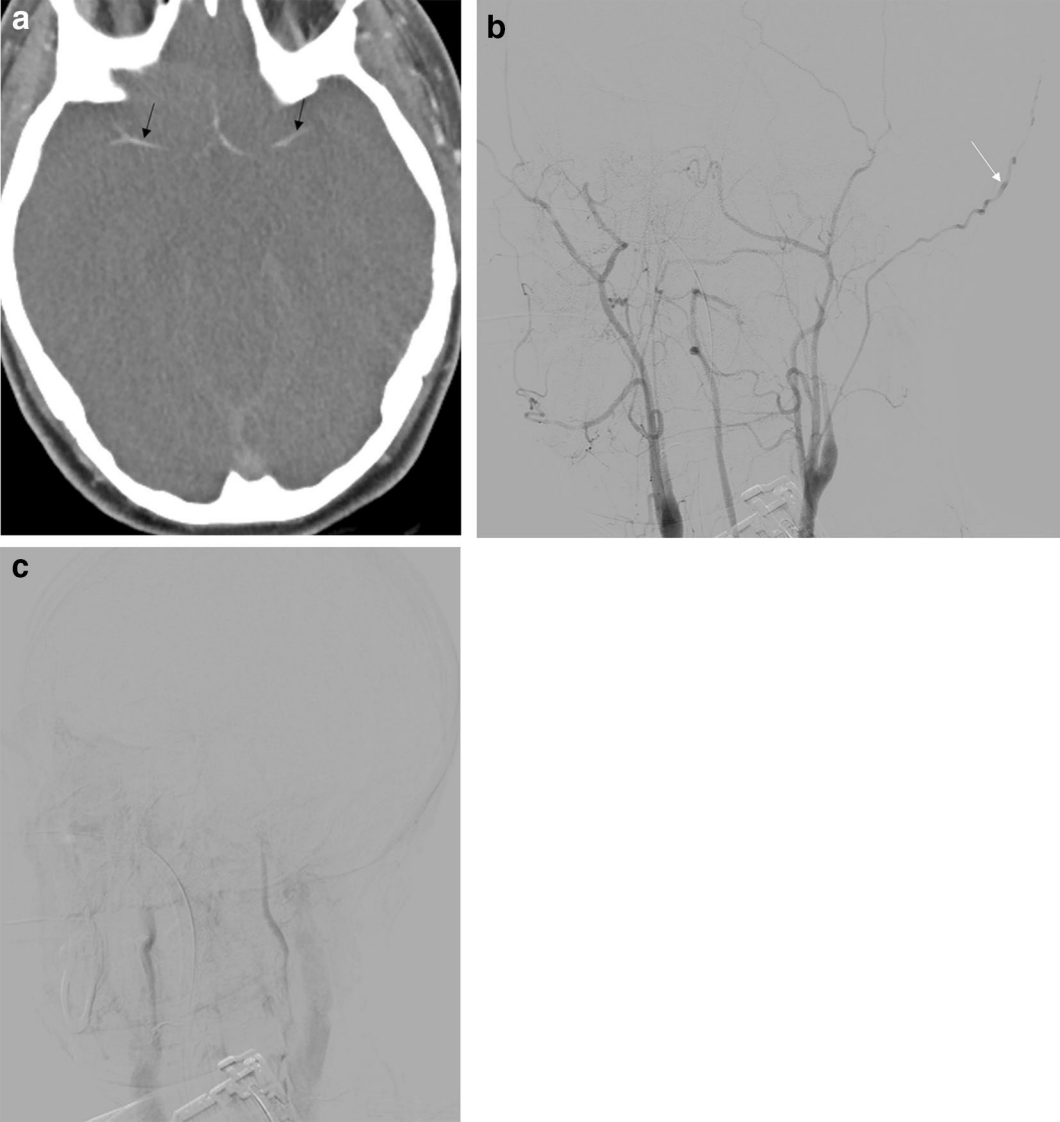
(a) Axial CTA (60 s phase) at the level of Circle of Willis, shows the stasis filling phenomenon, with opacification of proximal intracranial vessels (black arrows). (b) In the same patient, Digital Subtraction Angiography in arterial phase (AP view), shows no intracranial flow, while extracranial arteries are patent (white arrow). (c) In the same patient, Digital Subtraction Angiography in venous phase (AP view), shows no venous outflow.
